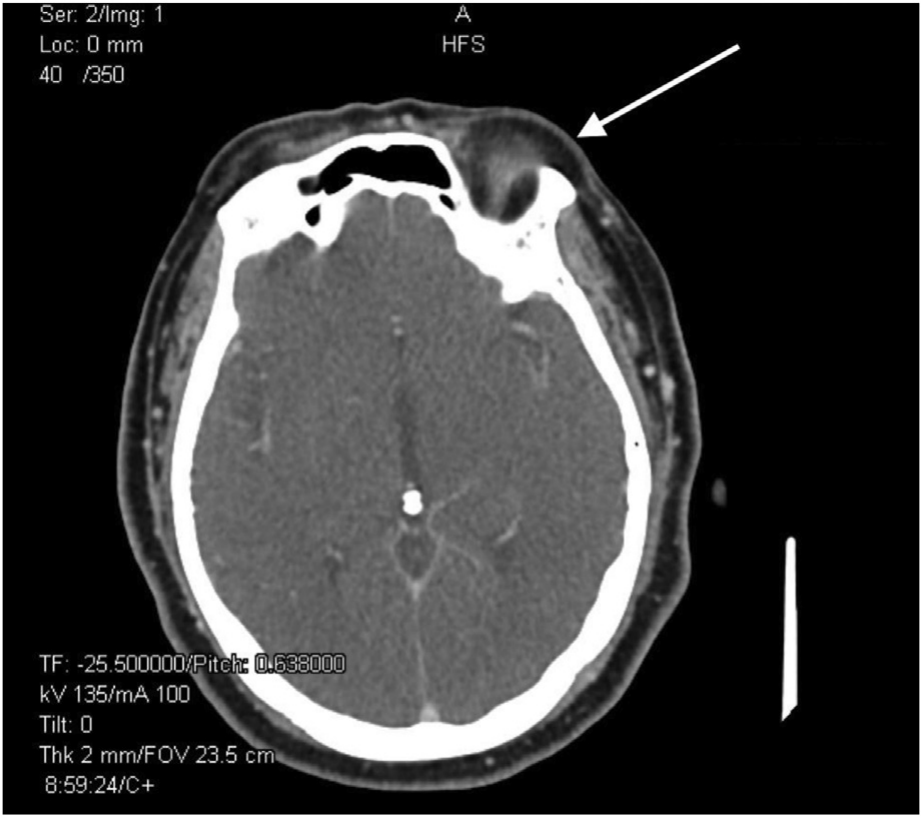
Axial image of computed tomography scan displayed a 2.6 cm transversely by 1.7 cm anteroposteriorly mass arising at the anterior aspect of the *right* parotid gland, extending to the subcutaneous region compatible with lymph node metastasis (*arrow* points to mass).
